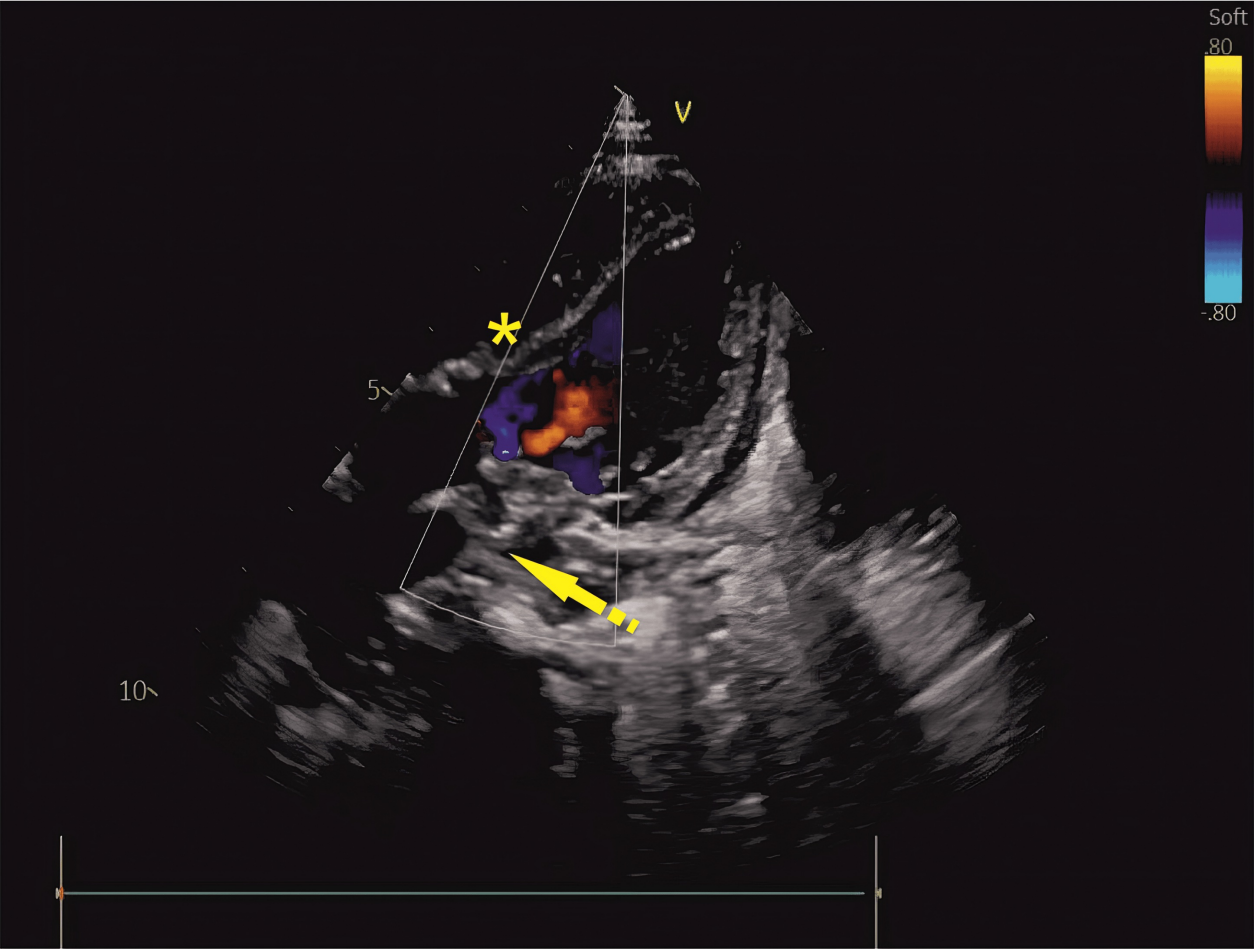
Two-dimensional echocardiography, apical four-chamber view The arrow indicates the left atrium, with a discrete echogenic mass abutting the mitral valve leaflet. The asterisk (*) denotes the left atrial cavity. Image Credits: Dr. Lamak Kadiyani
